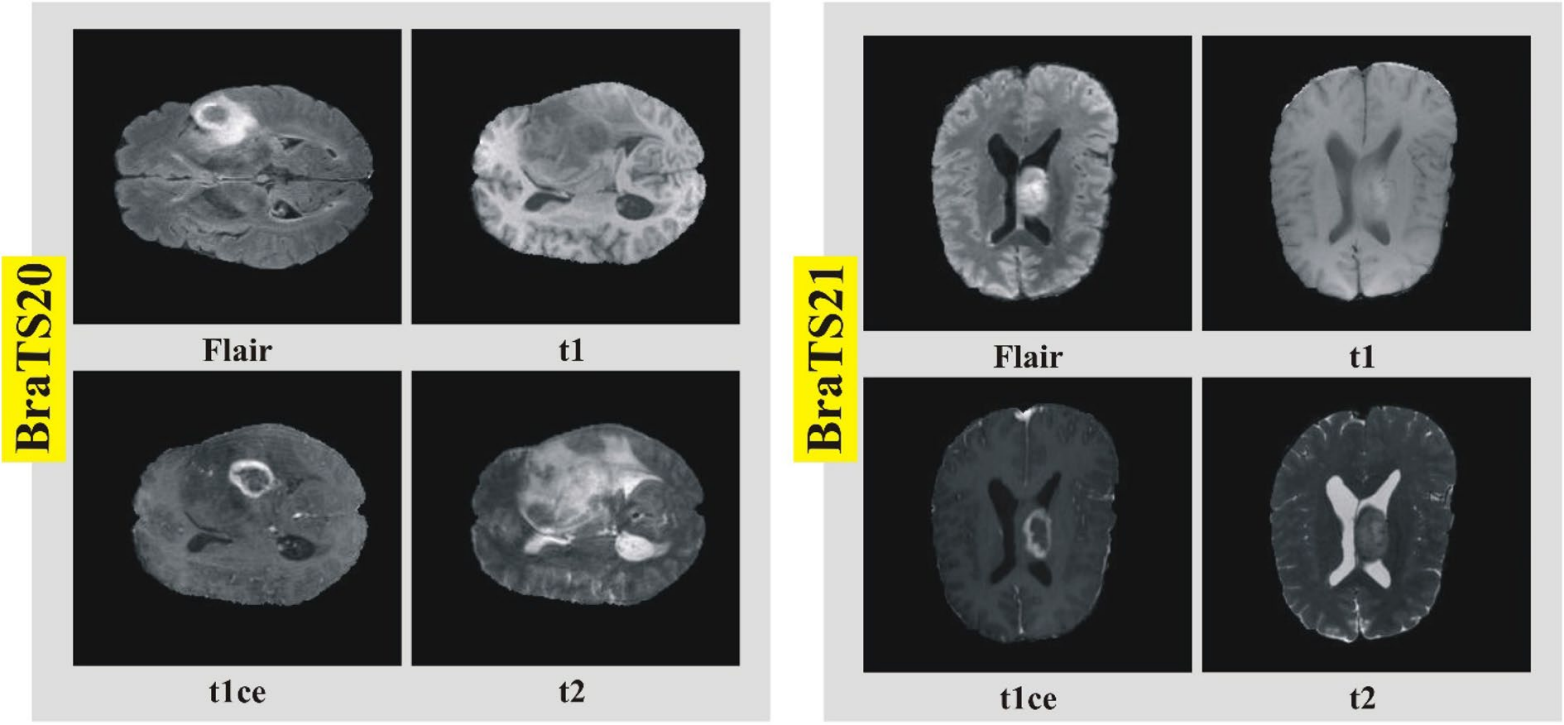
Sample MRI scans of brain tumor modalities for selected datasets.
